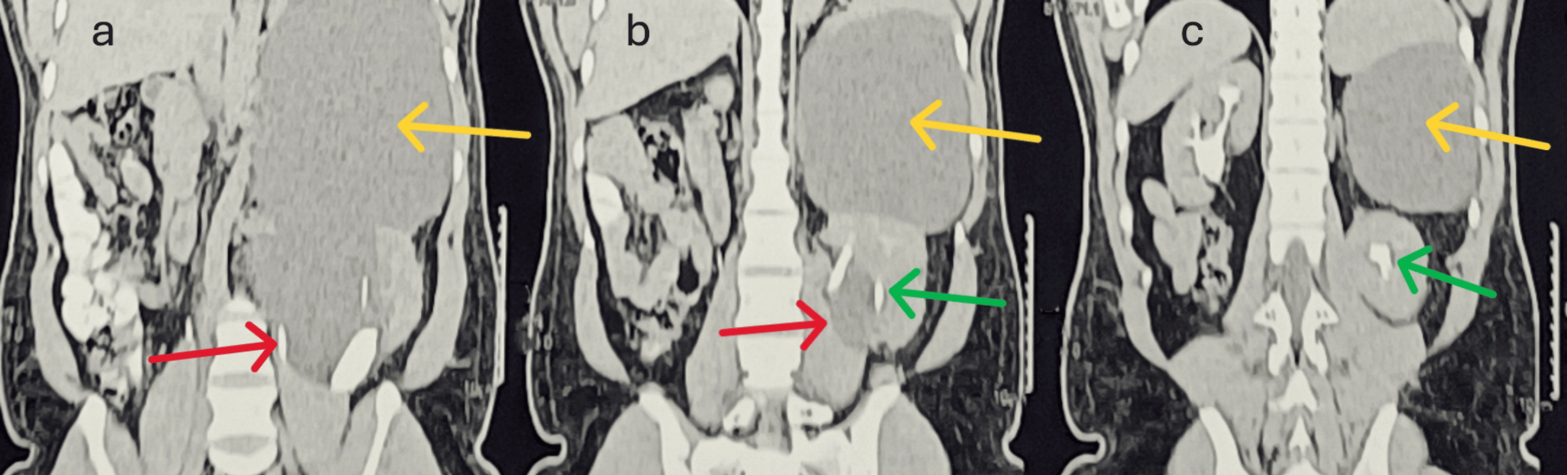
Coronal sections of CT demonstrating the origin and extensions of the parapelvic cyst. (a-c) The origin of the parapelvic cyst from the hilum (marked by red arrows in a and b) of the kidney and the superior extensions (marked by yellow arrows) of the parapelvic cyst pushing the kidney downwards. (b, c) Contrast opacification in the excretory phase, and infundibular splaying of the collecting system (marked by green arrows) due to the parapelvic cyst.
